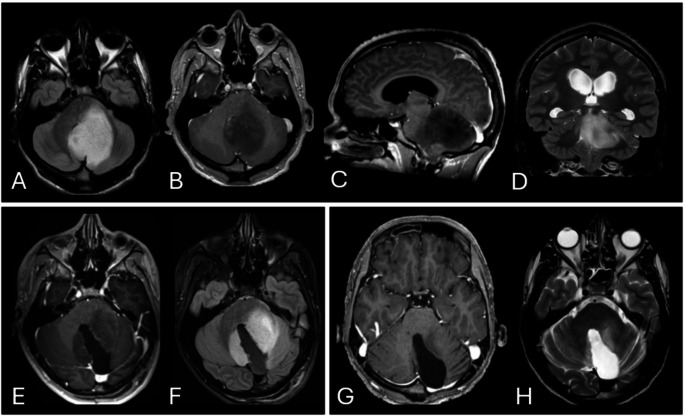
Preoperative (A, B, C & D), one-year postoperative (E & F), and two-year postoperative Brain MRI. (G & H). A preoperative axial T2-FLAIR MRI. B preoperative axial contrast-enhanced (CI) T1-weighted imaging (WI) MRI. C preoperative sagittal CI T1-WI MRI. D preoperative coronal T2-WI MRI. E one-year postoperative axial CI -T1-WI MRI. F one-year postoperative axial T2-FLAIR MRI. G two-year postoperative axial CI T1-WI MRI. H two-year postoperative axial T2-WI MRI
